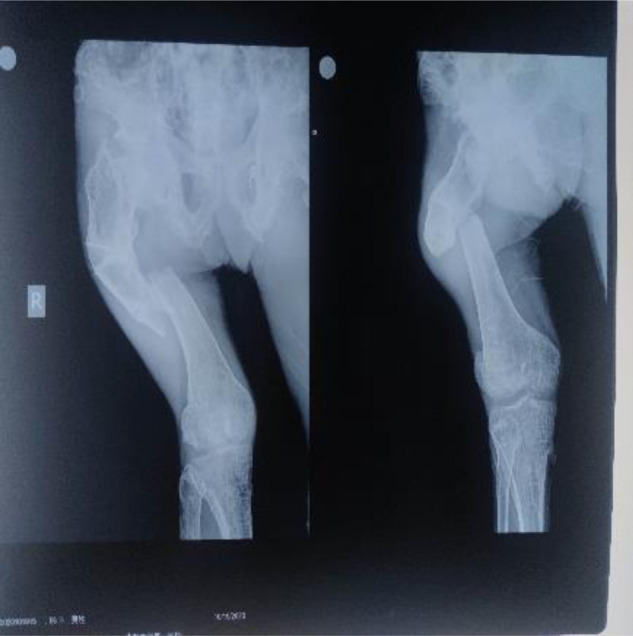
X-ray of the patient on admission.
